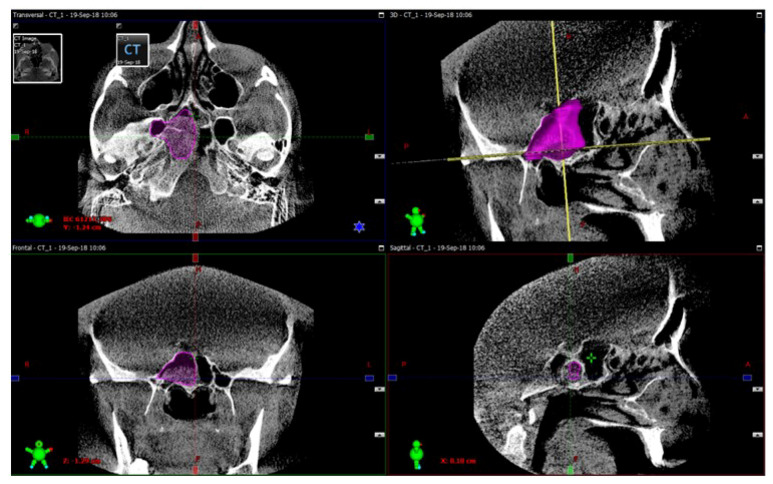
Sphenoid sinus volume measurement procedure based on computed tomography (CT) scanning followed by 3D reconstruction. The opacification of the sphenoid sinus is marked in purple. The exemplified opacified sinus volume highlighted by violet in the figure equals 9.4 cm^3^. The green color denotes the scan area; the red color marks isocenter position; the blue color is horizontal axis; while the yellow line denotes the head anatomical axis.
